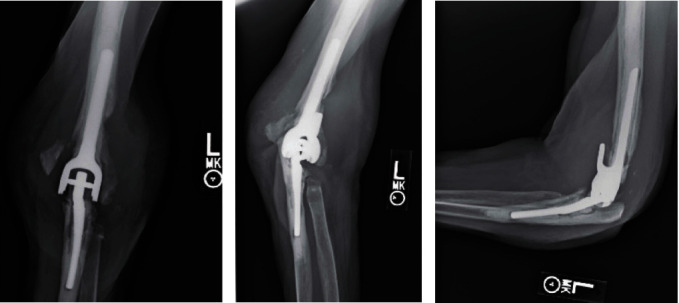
(a–c) Preoperative X-ray imaging of the operative left elbow showing prosthetic loosening and periprosthetic fracture. (a) AP imaging of the left elbow with new periprosthetic fracture of the medial epicondyle and significant soft tissue swelling. (b) Oblique imaging of the left elbow again demonstrating a periprosthetic fracture of the medial epicondyle and lucency around the lateral aspect of the humeral implant. (c) Lateral X-ray of the left elbow demonstrating both ulnar and humeral component lucency concerning for infection.
